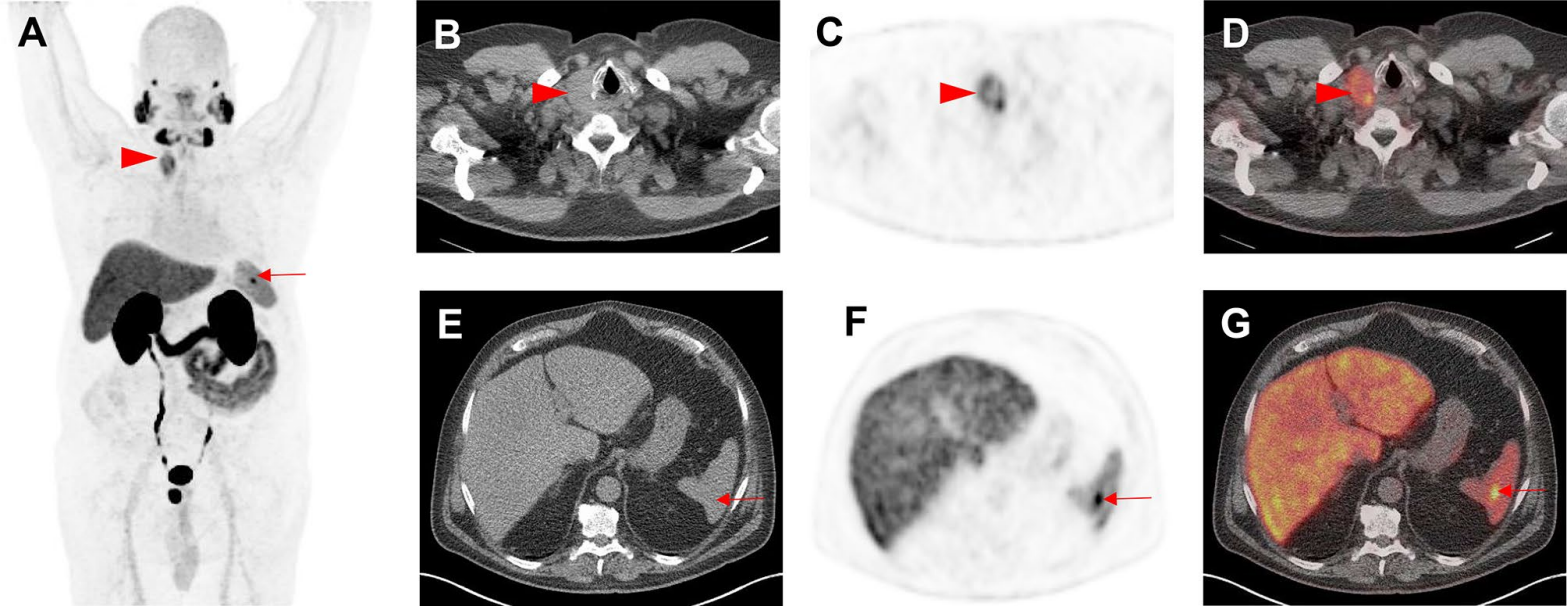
Indeterminate findings on prostate-specific membrane antigen (PSMA)-targeted positron emission tomography (PET)/computed tomography (CT). A 62-year-old man with history of biochemical recurrence undergoing [^18^F]-DCFPyL PET/CT. a Whole-body maximum intensity projection image demonstrates multiple foci of radiotracer uptake (red arrowhead and arrow). b Axial CT, c axial PET, and d axial PET/CT demonstrate intense radiotracer in a thyroid nodule (red arrowheads), which was classified as PSMA-RADS-3C and further workup (biopsy) was recommended. Subsequent biopsy yielded papillary thyroid carcinoma. e Axial CT, f axial PET, and g axial PET/CT show focal, intense radiotracer uptake in the spleen (red arrows). This was classified by an experienced reader as a likely inflammatory finding that was not suspicious for either prostate cancer or another malignancy and was labeled as PSMA-RADS-2. The overall scan score for this patient was, therefore, PSMA-RADS-3C
